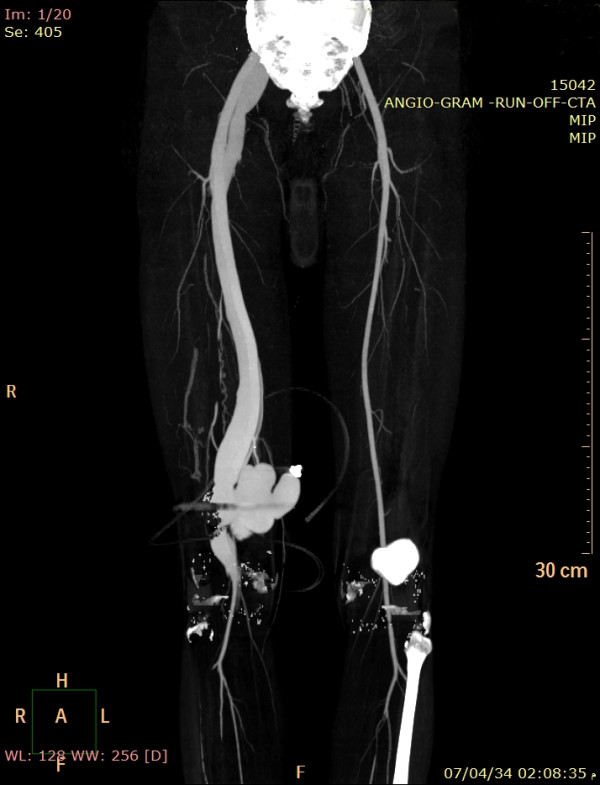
CT angiography showing a Femoropopliteal Pseudoaneurysm with dilated deep veins. Bullet shrapnels seen around pseudoaneurysm.
